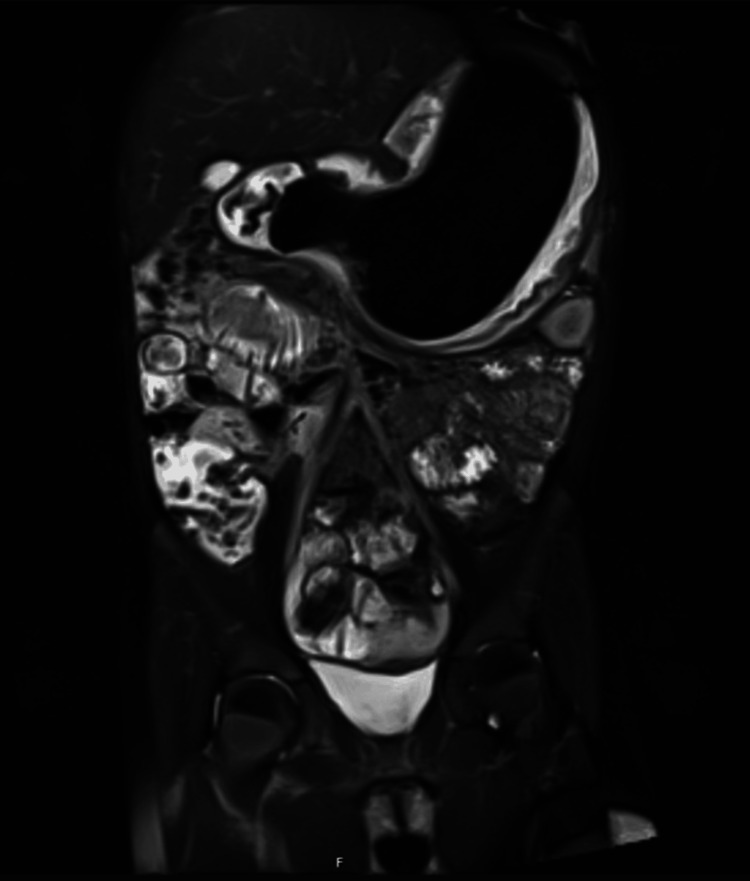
Magnetic resonance enterography (coronal T2-weighted image) demonstrating a large, well-defined intragastric filling defect that appears markedly hypointense The mass measures approximately 15–16 cm in length and extends into the proximal duodenum.
